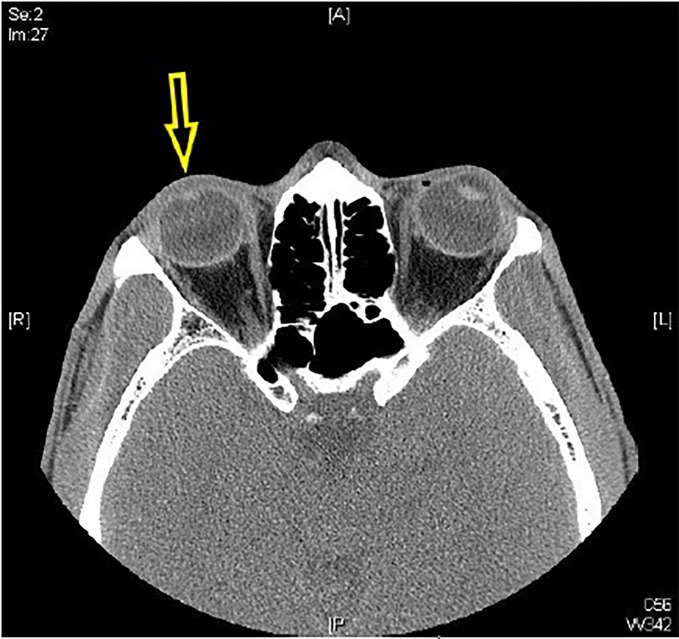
Computed tomography of the orbits, axial view, revealing right flat anterior chamber (yellow arrow) with iris abutting the cornea.
